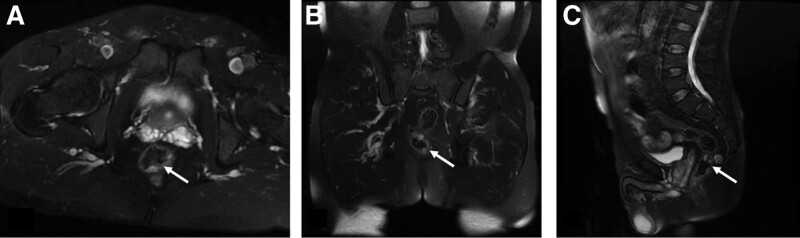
Post-treatment MRI image (T2W1): after treatment, the lesion of cavernous hemangioma in the lower rectal was significantly reduced, and the signal intensity was reduced (white arrow).
